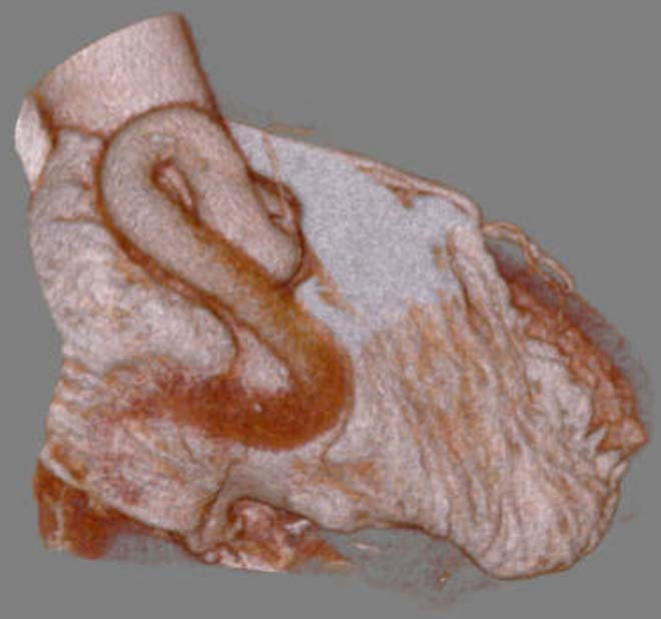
Postoperative coronary computed tomography shows no blood flow into the coronary aneurysm.
